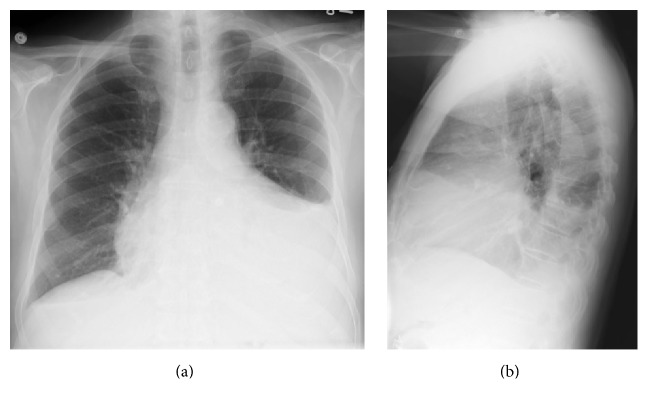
Conventional chest radiograph on the day of admission. The images show an enlarged cardiac silhouette, bibasilar opacities, and left-sided pleural effusion.
